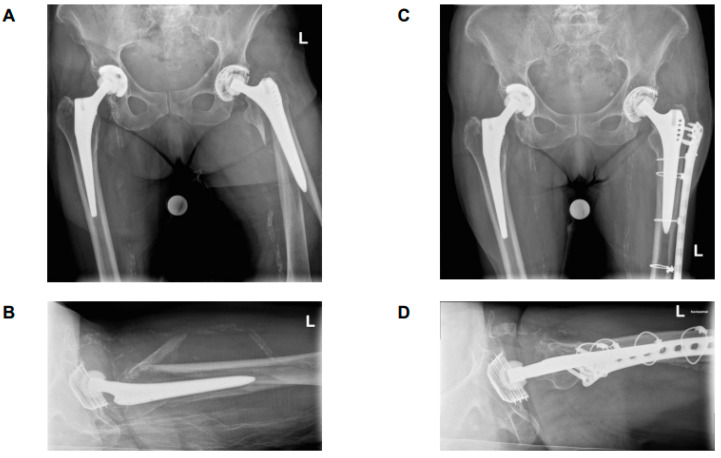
Antero-posterior and axial X-rays. (A,B) preoperatively; (C,D) 18-week-follow-up. A 93-year-old men with PPF, left, ORIF, excellent functional outcome. By definition, the stem is loose, but as the greater trochanter is still attached to the prosthesis, the patient has no limb and no stem subsidence.
